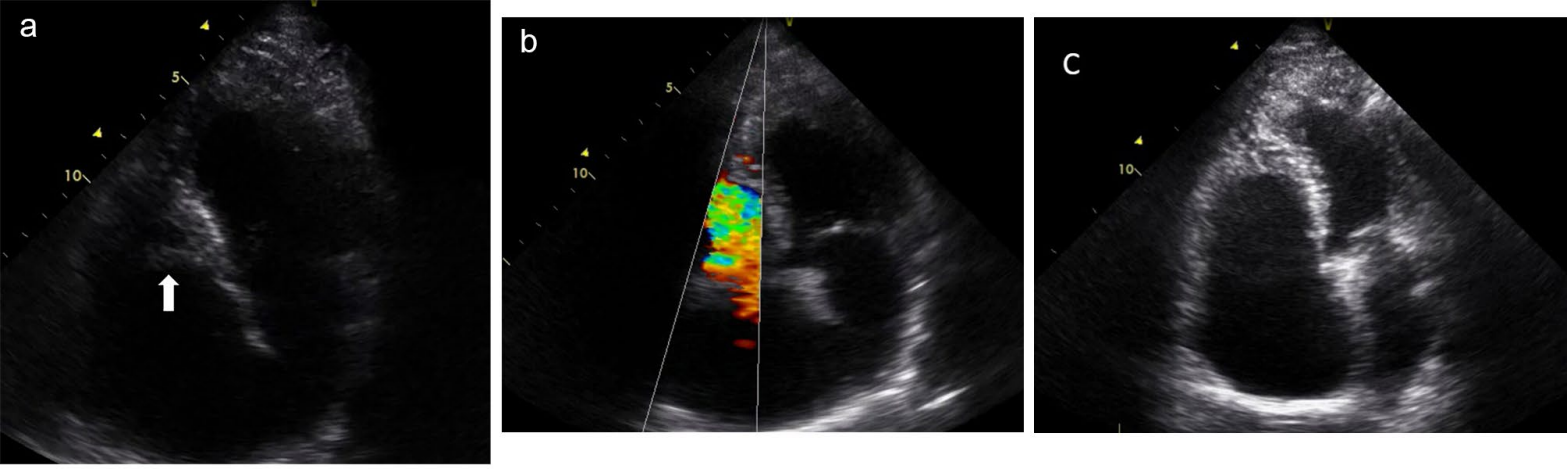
Apical 4-chamber view. Severe thickening of tricuspid valves with extension to the RV free wall is seen (A, arrow), resulting in an increased gradient and severe tricuspid stenosis (B). However, a follow-up echocardiogram two months later shows no evidence of tricuspid valve thickening and stenosis (C)
